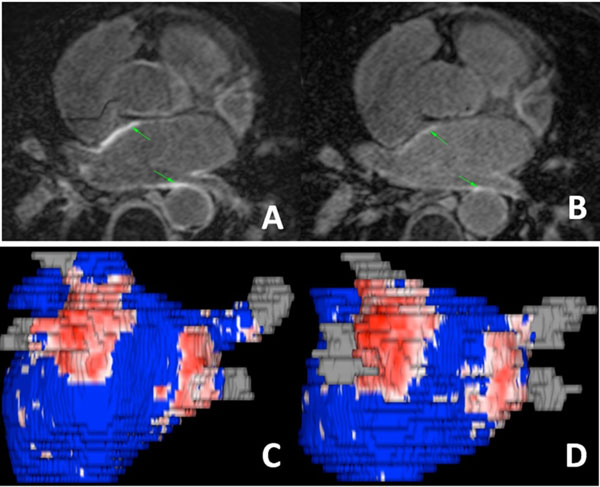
Scar detected by LGE-MRI performed 3 and 18 months post ablation.
